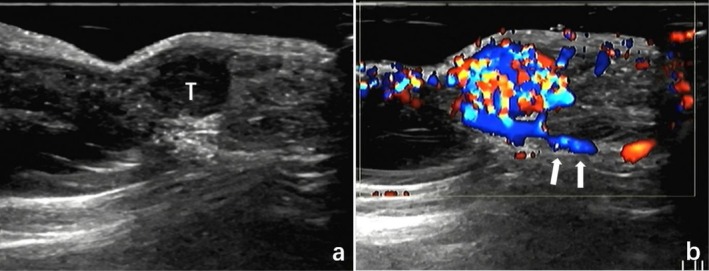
Extradigital glomus tumor in a 52‐year‐old female (left fourth digital pulp). (a) Gray‐scale ultrasound image shows that the tumor (T) is located within the digital pulp muscle. (b) Color Doppler shows intralesional hypervascularity and the presence of “vascular stalk sign” (→).
